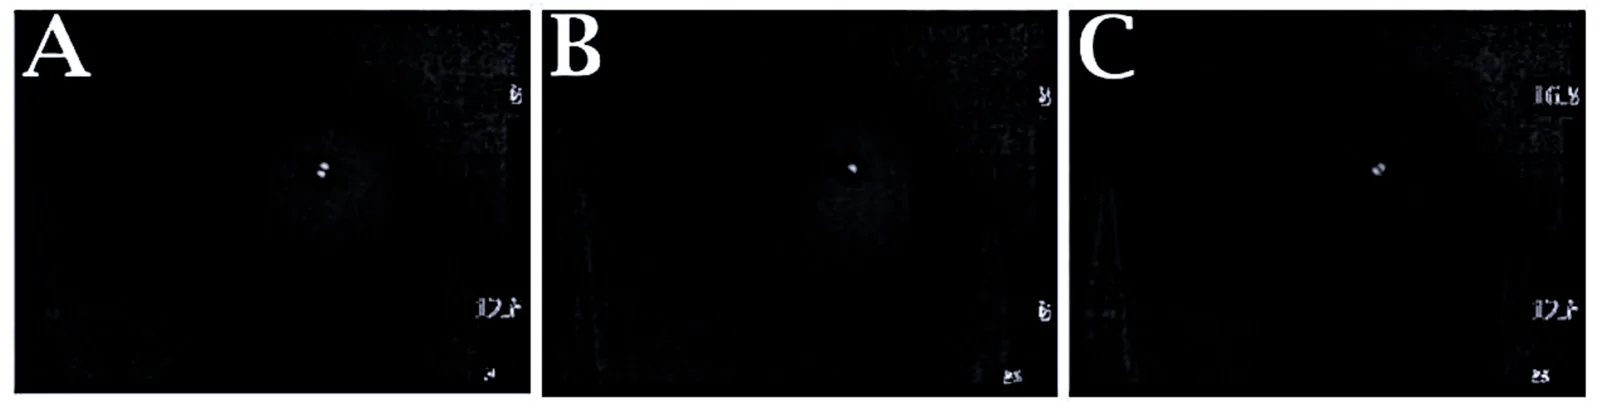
Registered digital subtraction images comparing untreated and laser-treated CT LUCIA IOLs: (A) after 5 Nd:YAG laser shots; (B) after 10 Nd:YAG laser shots; and (C) after 15 Nd:YAG laser shots. Bright regions indicate differences in transmitted light-spot position or intensity distribution.
